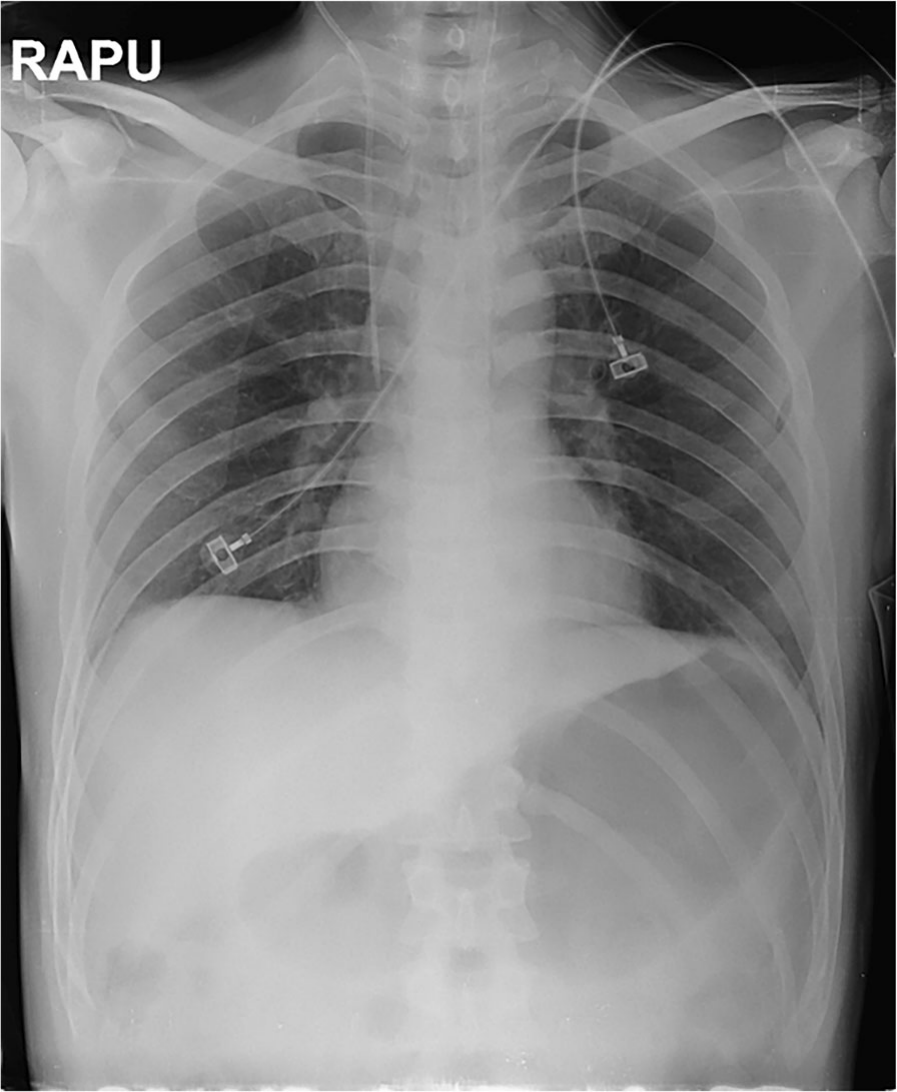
Chest X-ray at presentation
